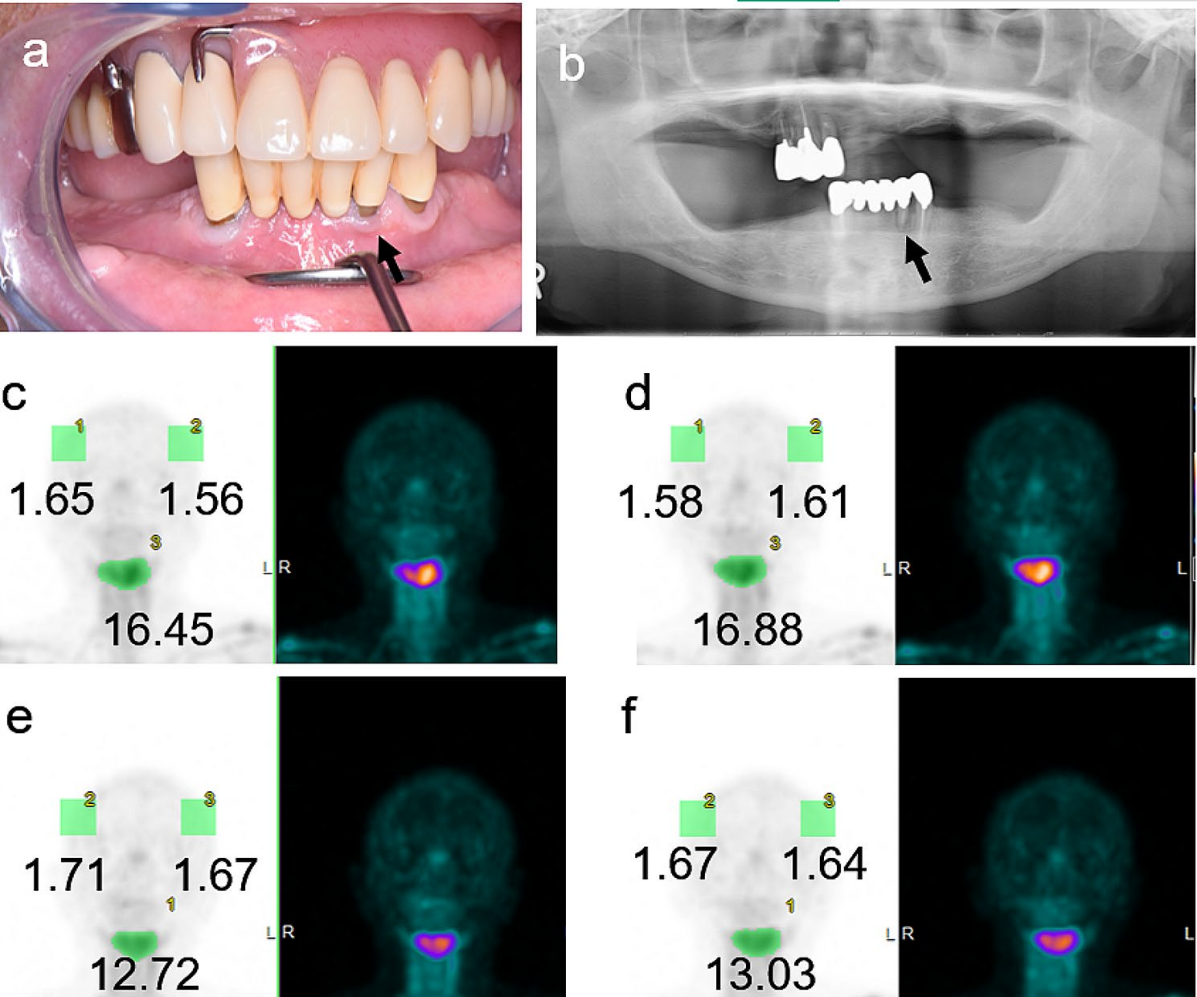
Depiction of a representative case (Patient 15). Patient 15 was a 61-year-old man who had been treated for bone metastasis of prostate cancer at our hospital. After being administered denosumab 15 times, the patient complained of mandibular pain while wearing a mandibular denture. No gingival swelling or bone exposure is observed in tooth number 32 (black arrow) (a). However, the tooth was tender on percussion. A panoramic radiograph shows a widening of the PDL space, suggestive of periodontitis, in tooth number 32 (black arrow) (b). The 2-h image for the first SPECT shows an SUVmax of 16.45 and strong accumulation in the midline of the mandible (c). In the 3-h image for the first SPECT, both the SUVmax of the parietal bones and SUVmax of the jawbone lesions are highly equivalent to the SUVmax of the corresponding 2-h images (d). In the second SPECT (2-h image), which was obtained 3 months later, improvement is observed in the lesion (SUVmax, 12.72). However, the SUVmax of the parietal bones has not changed (e). In the second SPECT, as in the first SPECT, the SUVmax of the parietal bones and the jawbone lesions show high equivalence in the comparative imaging evaluations of the 2-h and 3-h images (f). *PDL*, Periodontal ligament; *SPECT*, single-photon emission computed tomography; *SUVmax*, maximum standardized uptake value
